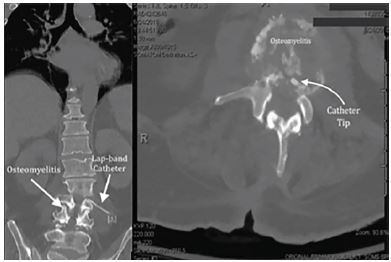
Computed tomography of the lumbar spine, (left) coronal view and (right) axial view demonstrating erosion of the laparoscopic gastric banding catheter into the spinal area and associated osteomyelitis.
